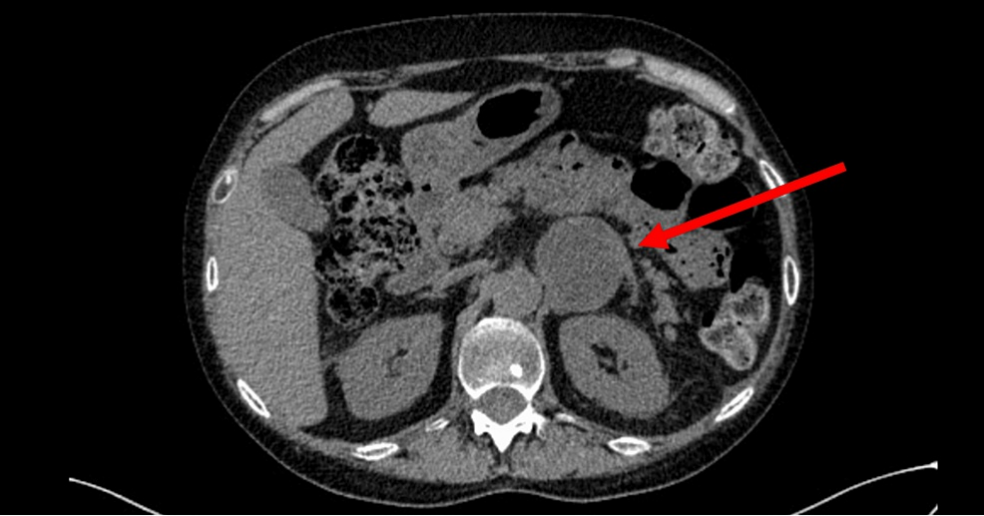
Abdominal CT revealing a 44 × 40 mm left adrenal mass (red arrow) CT, computerized tomography
